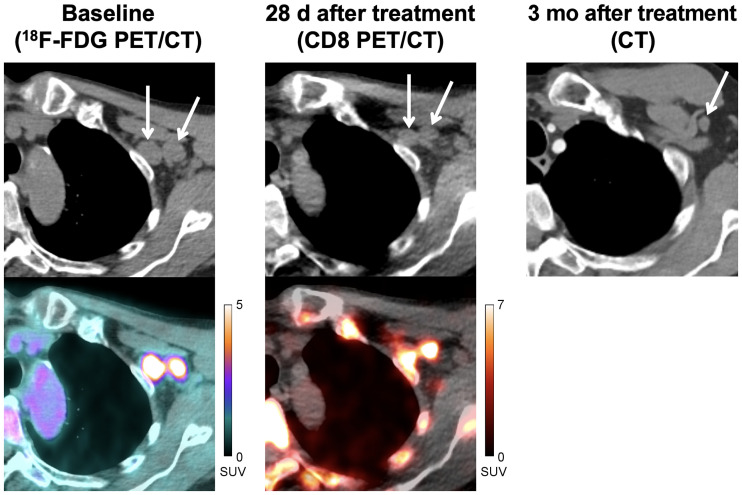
A 71-y-old man with locally advanced stage III melanoma treated with pembrolizumab. Baseline CT and fused ^18^F-FDG PET/CT images (left) demonstrate 2 ^18^F-FDG–avid metastases in left axilla (SUV_MAX_ = 10.0, medial node; SUV_MAX_ = 7.6, lateral node). CT and fused CD8 PET/CT images (middle) obtained at 28 d after start of immunotherapy demonstrate increased tracer activity in both metastases (SUV_MAX_ = 9.5, medial node; SUV_MAX_ = 10.0, lateral node), suggestive of tumor infiltration by CD8+ T cells. Follow-up imaging with contrast-enhanced CT (right) demonstrated complete response to therapy.
